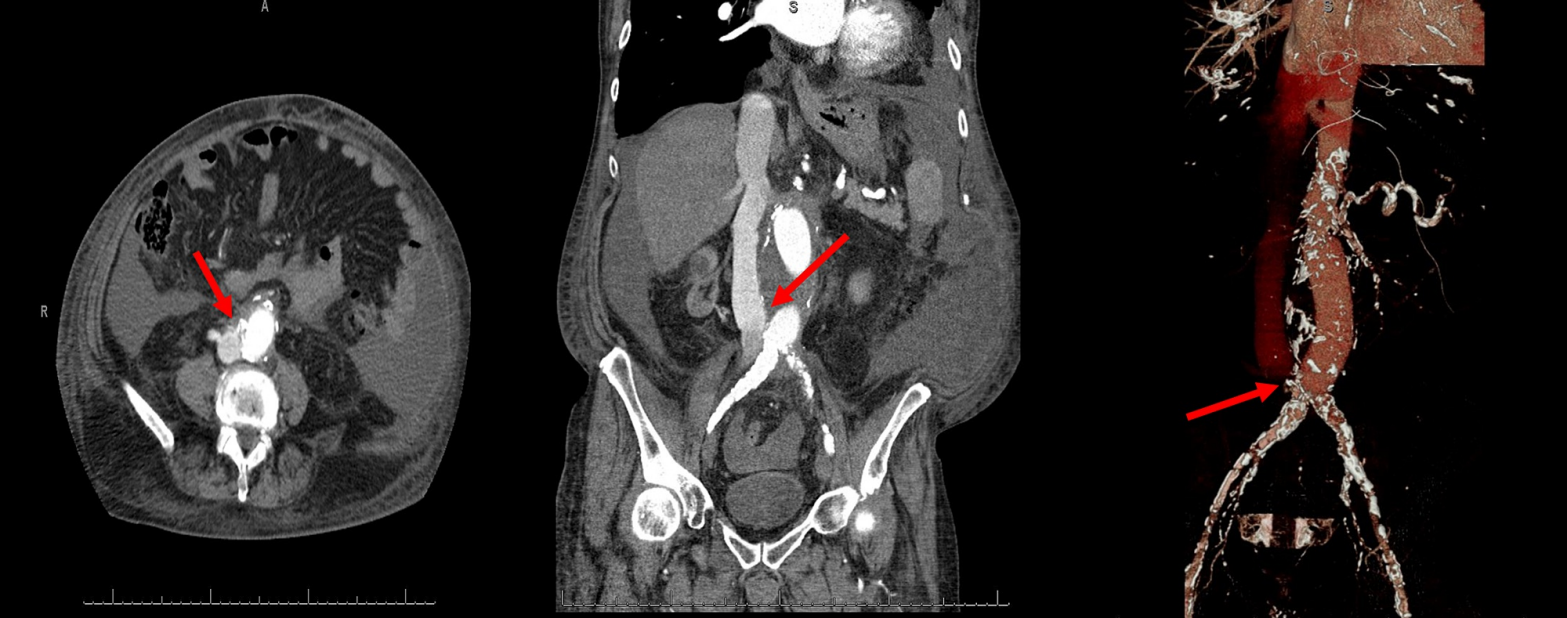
Computed tomographic angiography of the abdomen shows fistulous connection between AAA and inferior vena cava (red arrows) AAA: abdominal aortic aneurysm
